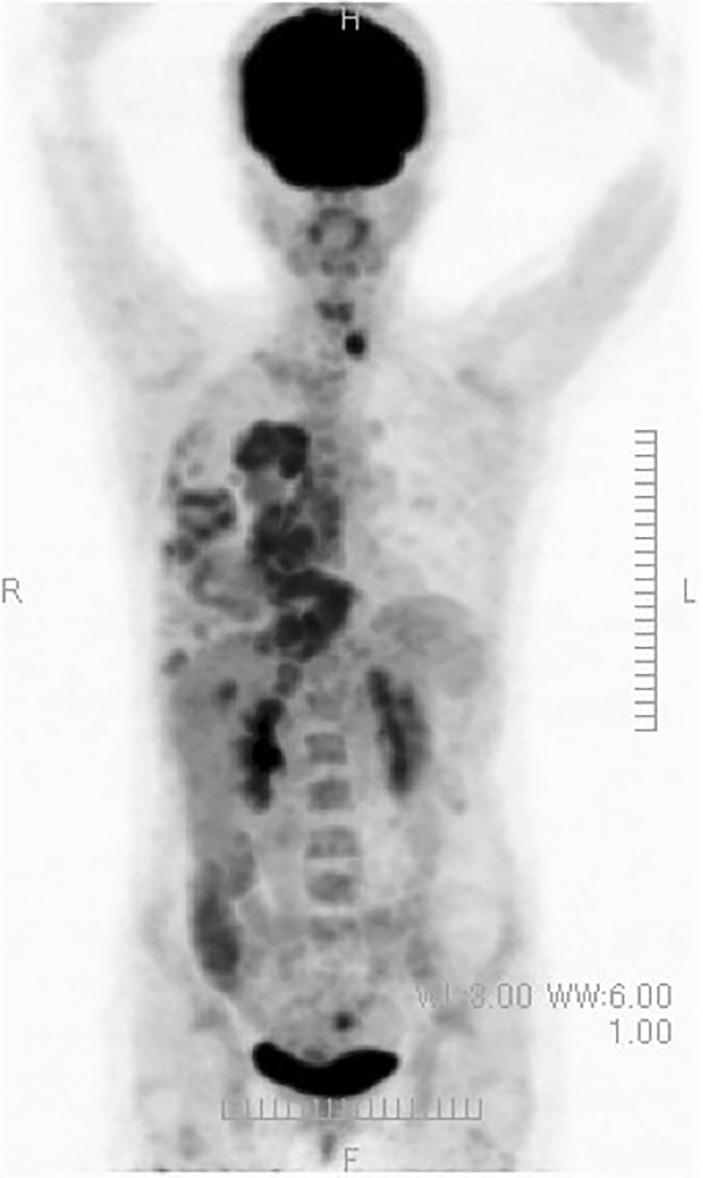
A PET/CT scan shows abnormal accumulations on her chest.
